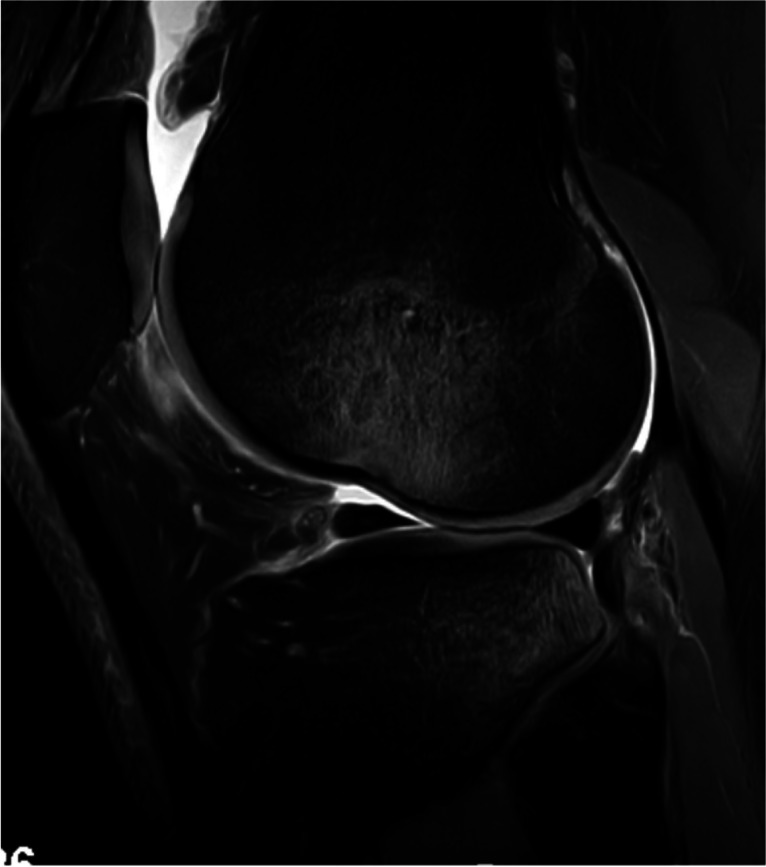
Pivot-shift type bone bruising as shown by the presence of bone marrow edema in the posterolateral area of the lateral tibial plateau and the central area of the lateral femoral condyle on sagittal proton density fat saturation magnetic resonance imaging sequence (left knee)
